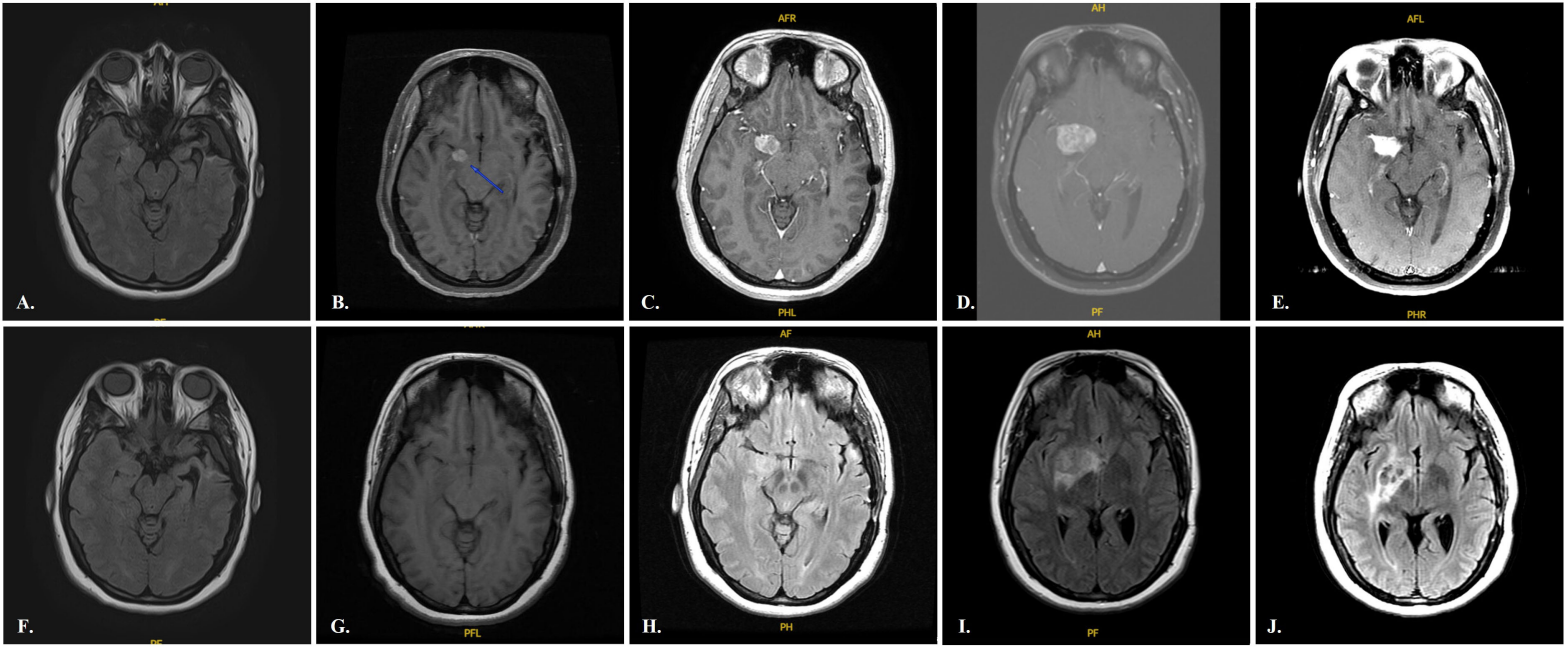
MRI of the brain showing stable post-surgical changes after first tumor resection, while on surveillance (A, F, 01/13/2014). New enhancing lesion develops in the medial right temporal lobe 7 x 11 x 12 mm (B, G, 03/09/2015), increasing in size and in surrounding vasogenic edema (C, H, 08/19/2015) and significantly continues to increase in size (D, I, 02/29/2016). Chemoradiation is started followed by maintenance cyclic temozolomide and the tumor shows shrinkage after 12 cycles of temozolomide (E, J, 09/26/2017).
